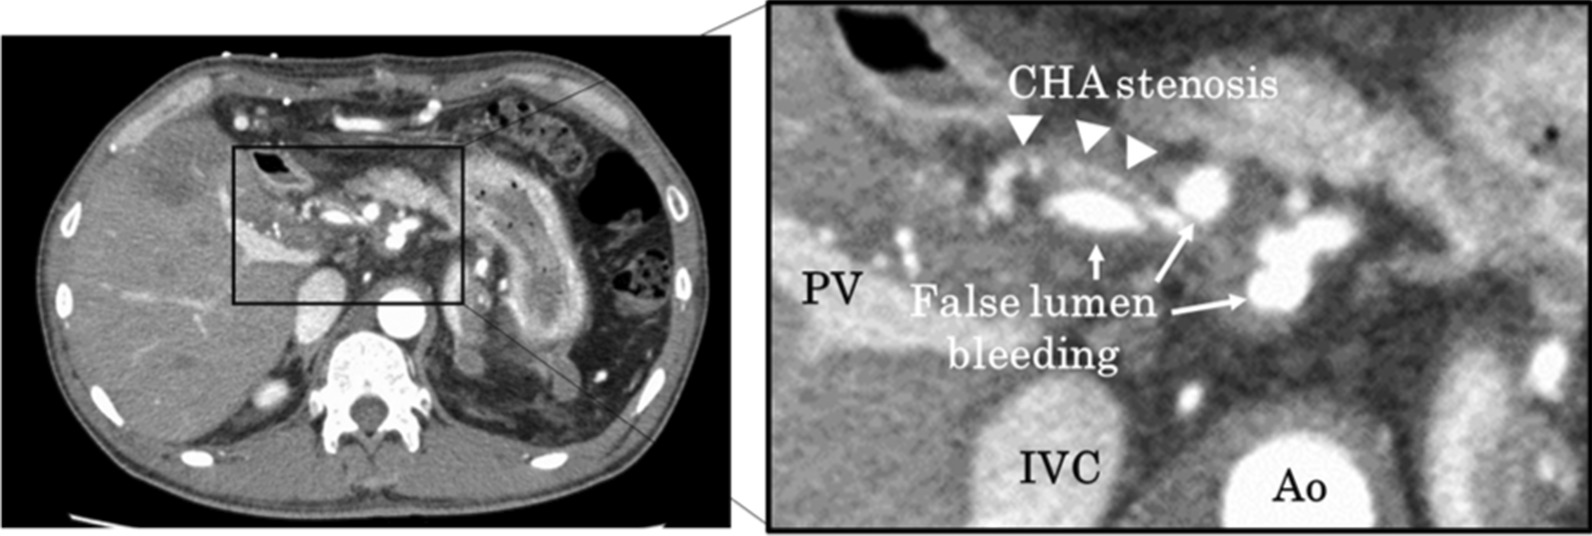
Contrast-enhanced CT in Case 1. The contrast-enhanced CT image showed narrowing of the common hepatic artery (CHA) and some bleeding in the false lumen; the bleeding appeared similar to an aneurysm. Abbreviations: PV, portal vein; IVC, inferior vena cava; Ao, aorta
